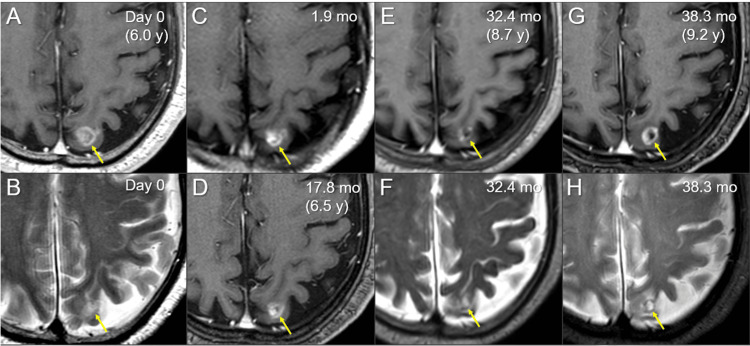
MRI findings before and after the initial stereotactic radiosurgery The images show axial CE-T1-WIs (A, C, D, E, G) and axial T2-WIs (EB, F, H); on the day (Day 0) of SRS (6.0 years [y] after the first-line chemotherapy) (A, B); at 1.9 months (mo) after SRS (C); at 17.8 months (D); at 32.4 months (E, F); and at 38.3 months (9.2 years after the first-line chemotherapy) (G, H) (A-H) These images are shown at the same magnification and coordinates under co-registration and fusions. Therefore, some images with thick slices are slightly blurry (B, C, E, F, H). (C, D) T2-WIs were unavailable at 1.9 and 17.8 months. (A-F) The lesion regressed gradually (arrows in A-D), leading to the nadir response (arrows in E, F) with minimal enhancing effects and surrounding edema. (G, H) At 38.3 months, the enhancing effects (arrow in G) increased significantly, and the configuration of the mass visible on T2-WIs (T2-mass, arrow in H) became clearer MRI: magnetic resonance imaging; CE: contrast-enhanced; WIs: weighted images; SRS: stereotactic radiosurgery
